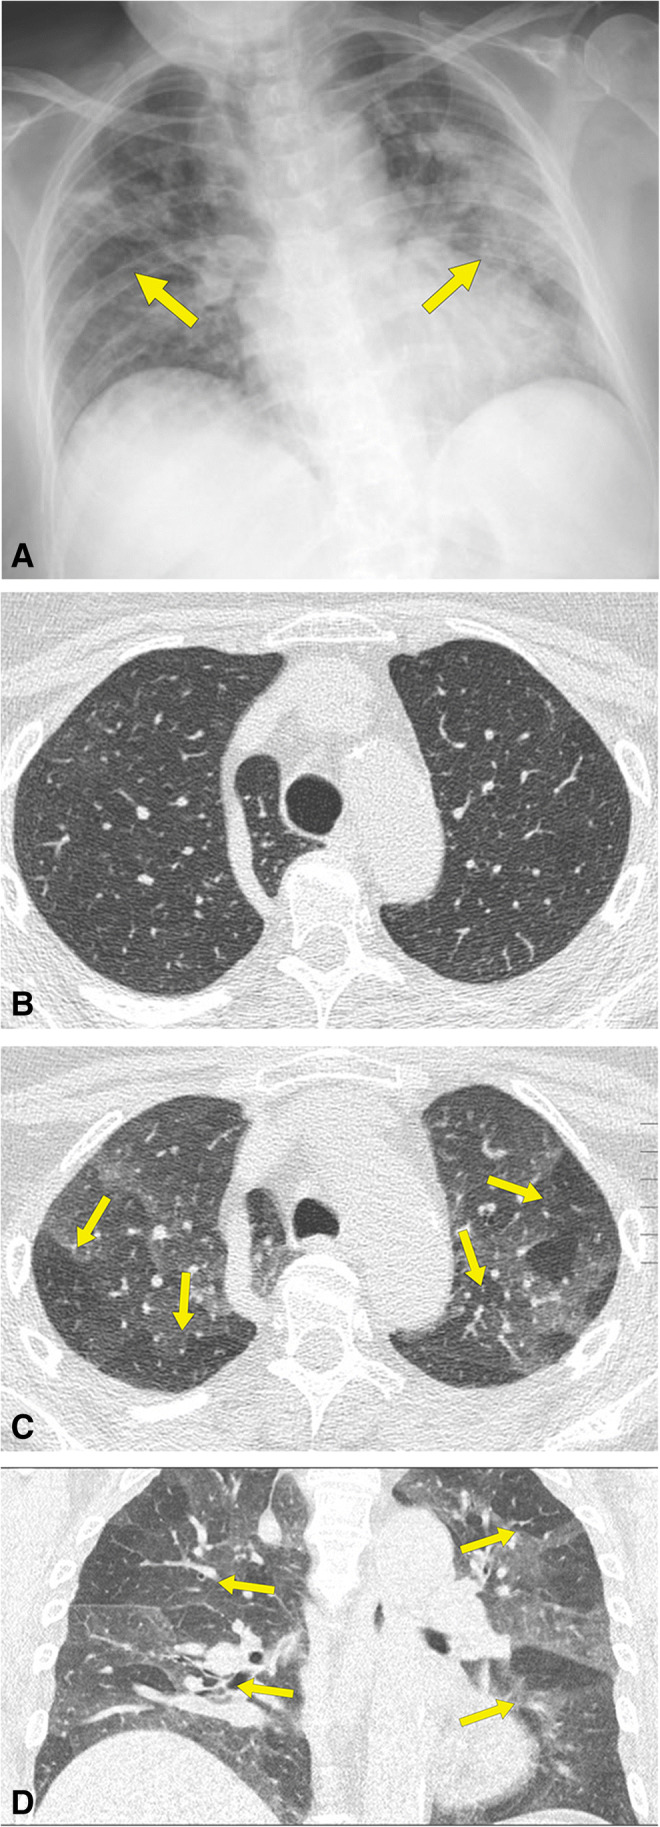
A 67-year-old nonsmoking man who had COVID-19. A Anteroposterior chest radiograph shows bilateral and multifocal areas of consolidation (arrows). B Inspiratory CT scan at follow-up examination, 65 days after dyspnea and shortness of breath shows no abnormalities. C Expiratory CT scan, obtained at same level as B, and D coronal multiplanar reformation image shows significant bilateral patchy areas of low attenuation due to air trapping (arrows)
